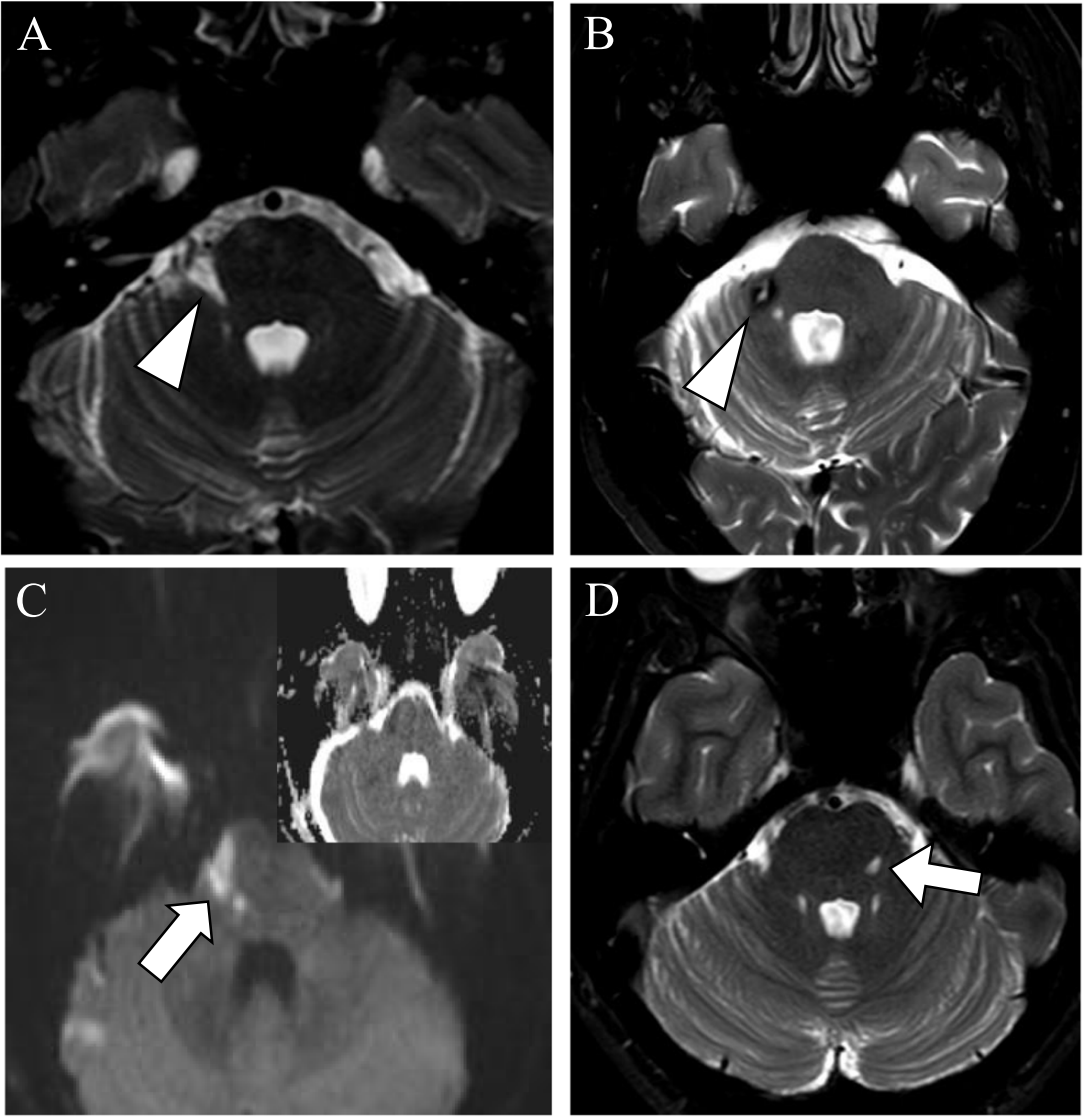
Postoperative MRIs of patients with stroke after microvascular decompression. Postoperative MRI of patients after MVD. (a) axial T2 DRIVE weighted sequence shows chronic infarction (arrowhead) in the right cerebellar peduncle of patient no 1 (Supplementary material B). (b) axial T2 DRIVE weighted sequence shows sequelae after hemorrhage (arrowhead) at the right cerebellar peduncle of patient no. 7. c) axial diffusion-weighted sequence shows subacute infarction (arrow) in the right side of the pons of patient no. 3. (d) axial T2 DRIVE weighted sequence shows chronic infarction (arrow) in the left side of the pons in patient no. 4
